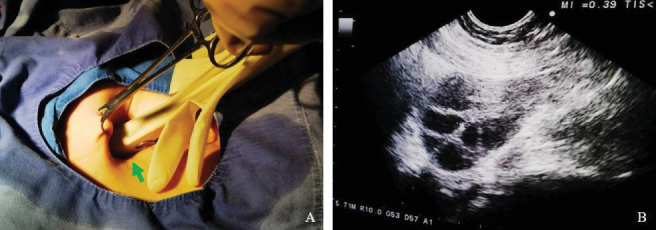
Transabdominal detection of the right ovary using a vaginal ultrasound probe. (A) Ultrasound probe positioned within the abdominal skin fold. (B) Clear ultrasound image of the right ovary.
